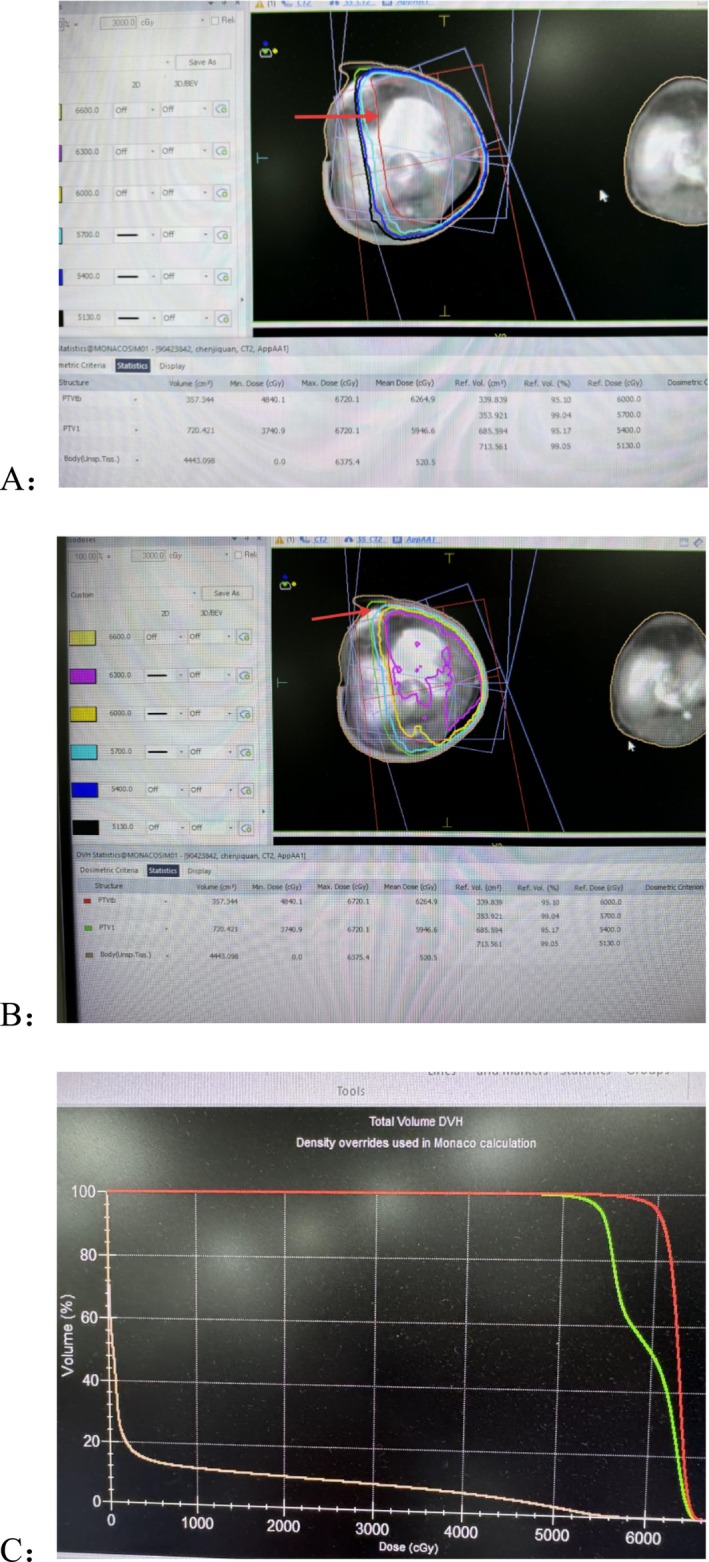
Axial computed tomography (CT) and dose distribution of postoperative radiotherapy plan for left femoral tumor (A, B). Axial CT image shows the multi‐field radiotherapy plan (red and blue beams), with colored isodose lines corresponding to different prescribed doses: 6300 cGy (magenta), 6000 cGy (yellow), 5700 cGy (cyan), 5400 cGy (blue), 5130 cGy (black). Arrow indicates planned target volume (PTVtb, red line, A) and clinical target volume (PTV1, green line, B). Dose‐volume histogram (DVH) statistical table: The average dose of the planned target volume (PTVtb) is 6264.9 cGy (95.10% of the volume has reached 6000 cGy), the average dose of PTV1 is 5946.6 cGy (95.17% of the volume has reached 5400 cGy), and the average dose of the skin tissue is 520.5 cGy. DVH curve (C): Total‐volume DVH generated via Monaco planning system's density override algorithm. The vertical axis represents volume percentage (Volume, %), and the horizontal axis represents radiation dose (Dose, cGy). Curves represent PTVtb (red), PTV1 (green), and normal skin (light brown).
